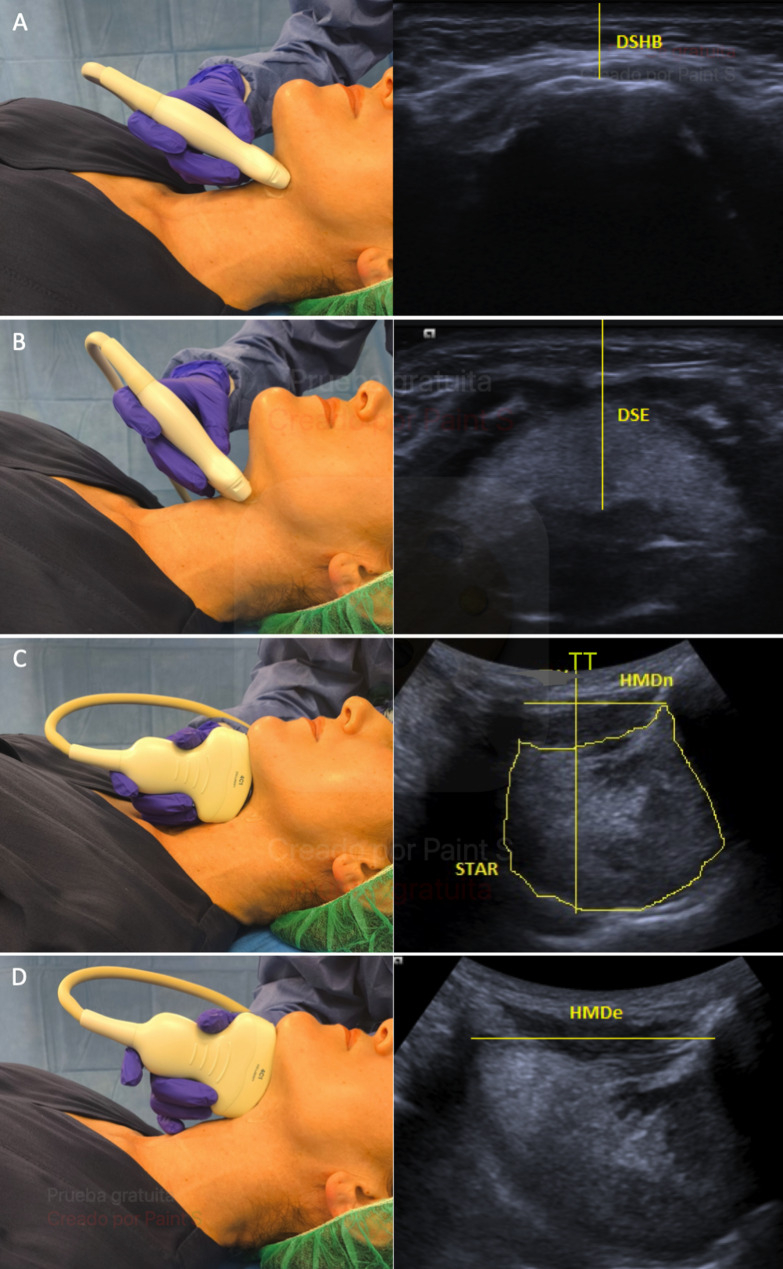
Airway ultrasound parameters used for machine learning-based prediction of videolaryngoscopy grade. Panel A Skin-to-hyoid bone distance (DSHB); Panel (B) Skin-to-epiglottis distance (DSE); Panel (C) Sagittal tongue area (STAR), tongue thickness (TT), and hyoid-to-mentum distance in neutral position (HMDn); Panel (D) Hyoid-to-mentum distance in extended position (HMDe). The hyoid-to-mentum ratio (HMDr) was calculated as HMDe divided by HMDn
